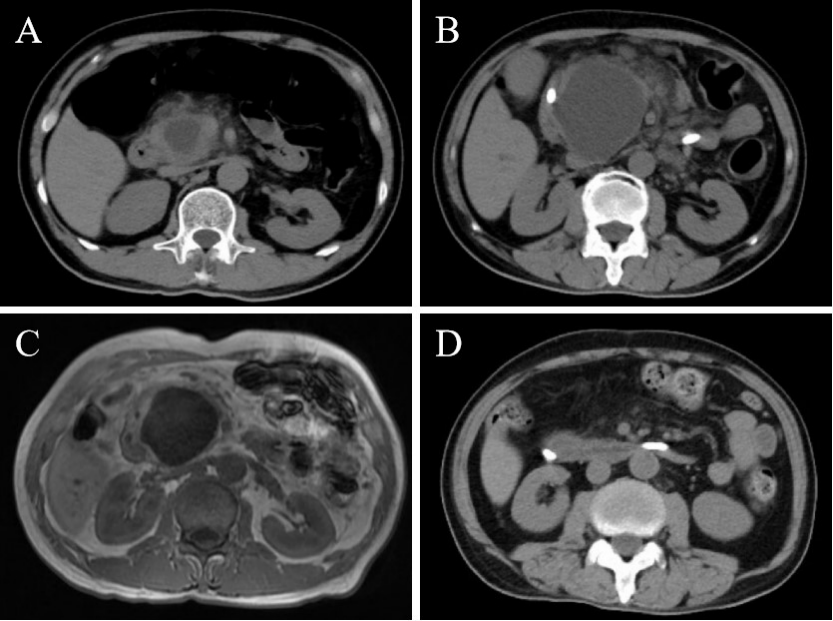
Images of the patient’s pancreas. Initial CT scan on admission (A) demonstrated a well-circumscribed cystic lesion (36 mm × 26 mm) within the pancreatic head, accompanied by peripancreatic exudation. CT scan after two weeks (B) showed progressive enlargement of the cyst to 67 mm × 49 mm, accompanied by dilation of the branch pancreatic ducts. Contrast-enhanced MRI (C) revealed hypointense on T1-weighted images and hyperintense on T2-weighted images within the pancreatic head, with no abnormal enhancement post-contrast administration. Follow-up CT scan after two weeks (D) demonstrated a significant reduction in cyst lesion size and resolution of peripancreatic exudation.
